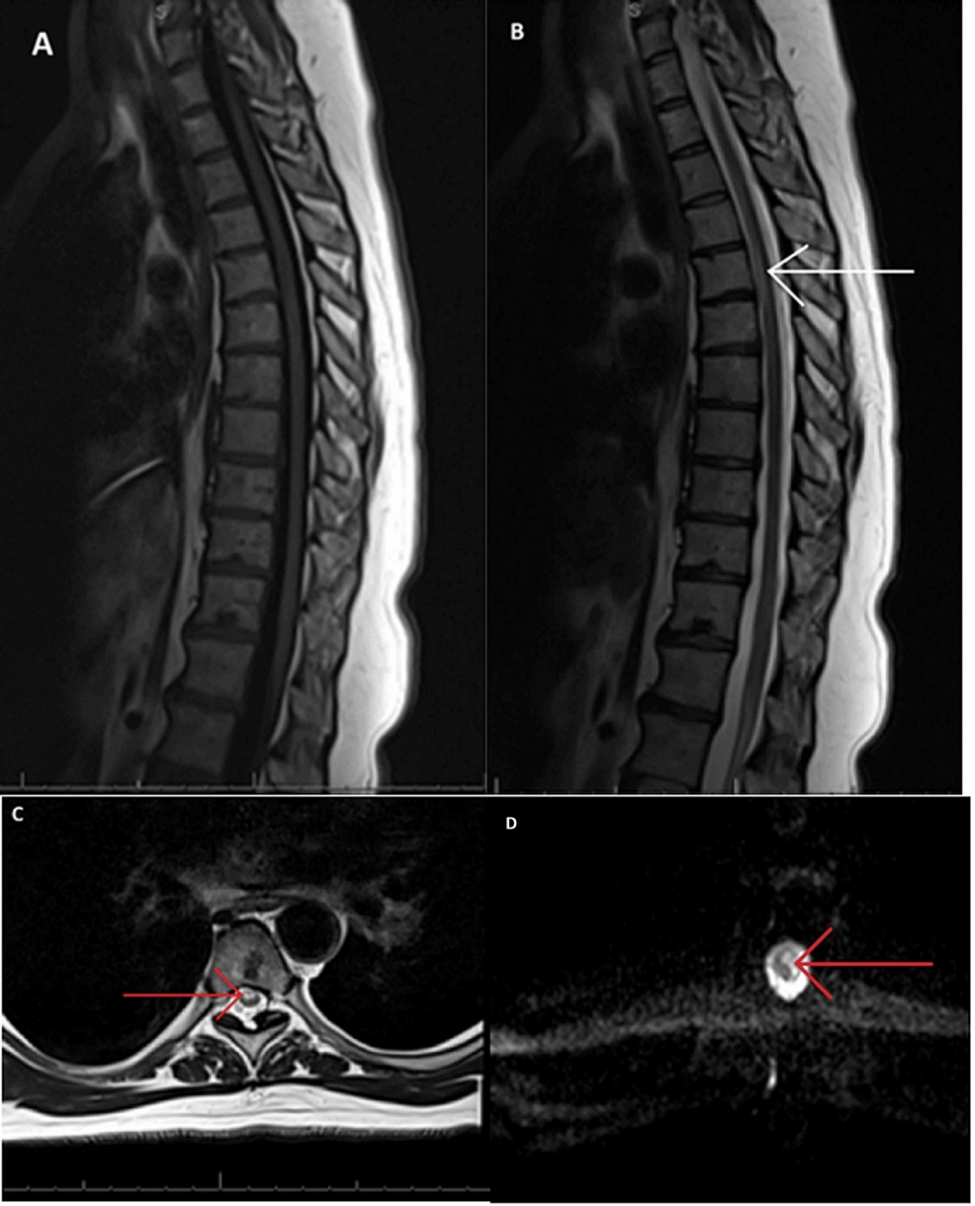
Magnetic resonance imaging of the thoracic spine. A) Sagittal T1 sequence was unremarkable. B) T2 sequence showing non-enhancing, linear hyperintense signal in the anterior bilateral spinal cord from T3-T5 without appreciable cord expansion or atrophy. C) Axial T2 sequence at T4 showing hyperintense signal in the anterior bilateral spinal cord. D) Axial diffusion-weighted imaging sequence at T4 showing diffusion restriction in the spine.
